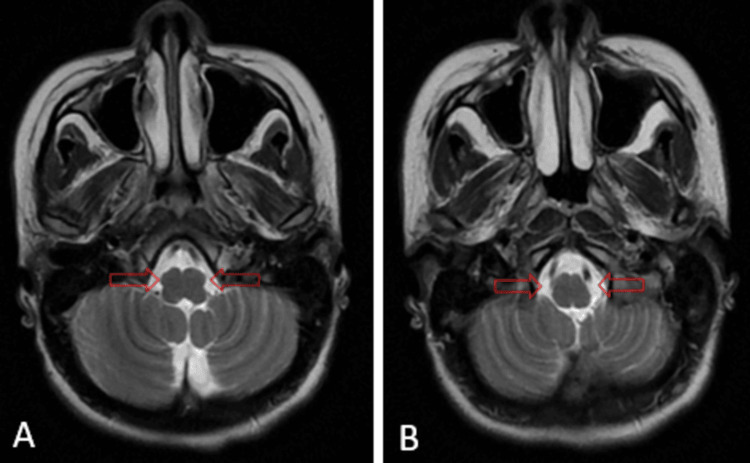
(A, B) Magnetic resonance imaging. Axial T2-weighted imaging did not show an increased signal of the inferior olivary nucleus (red arrows) or enhancement following gadolinium injection.
